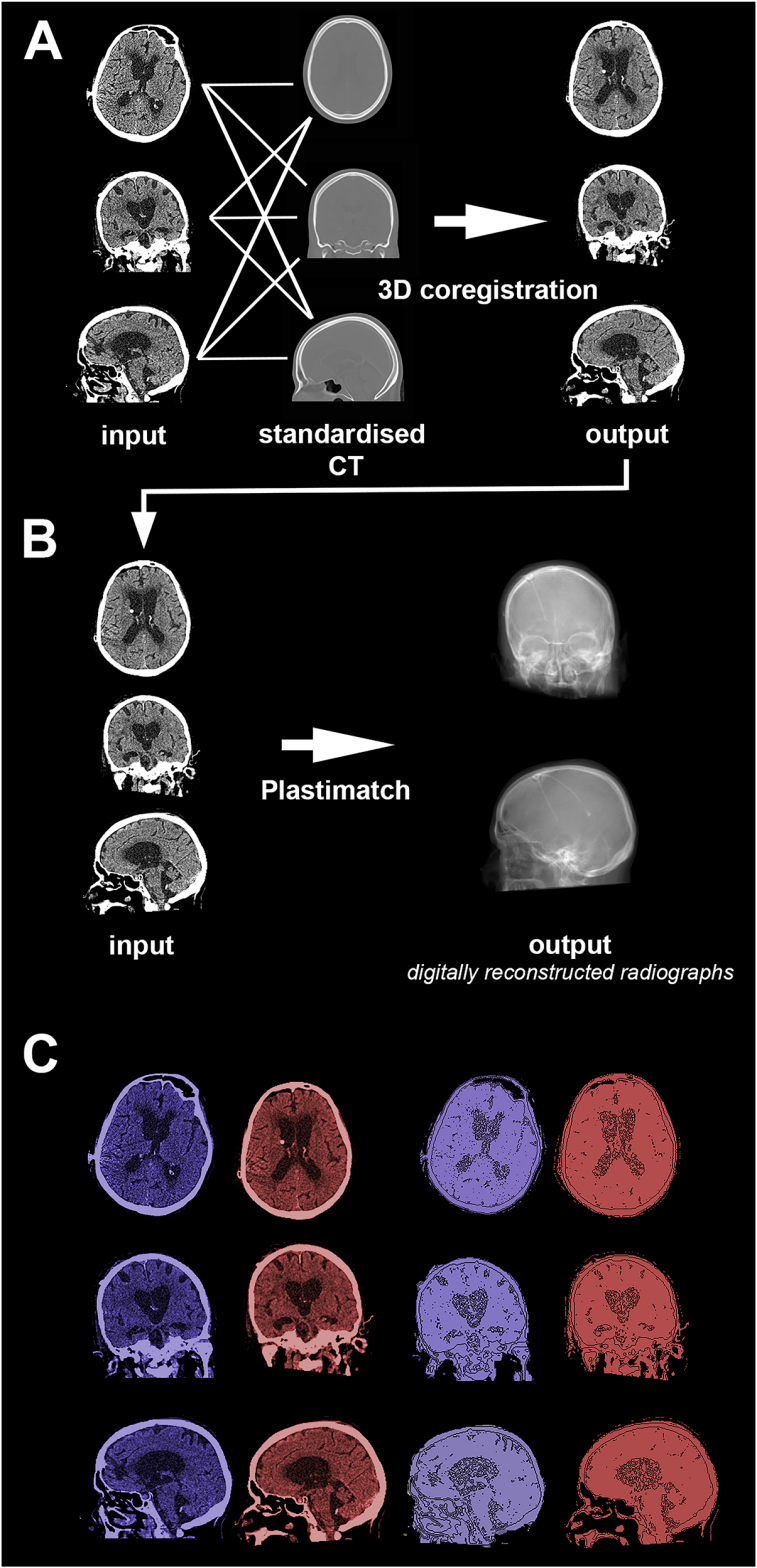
Preprocessing for model 1. A Step1: registration of a windowed CT scan to a standardized CT scan. B Step 2: creating a digitally reconstructed radiograph from the now standardized CT scan. C Comparison of input CT (blue) vs same CT after coregistration (red)
